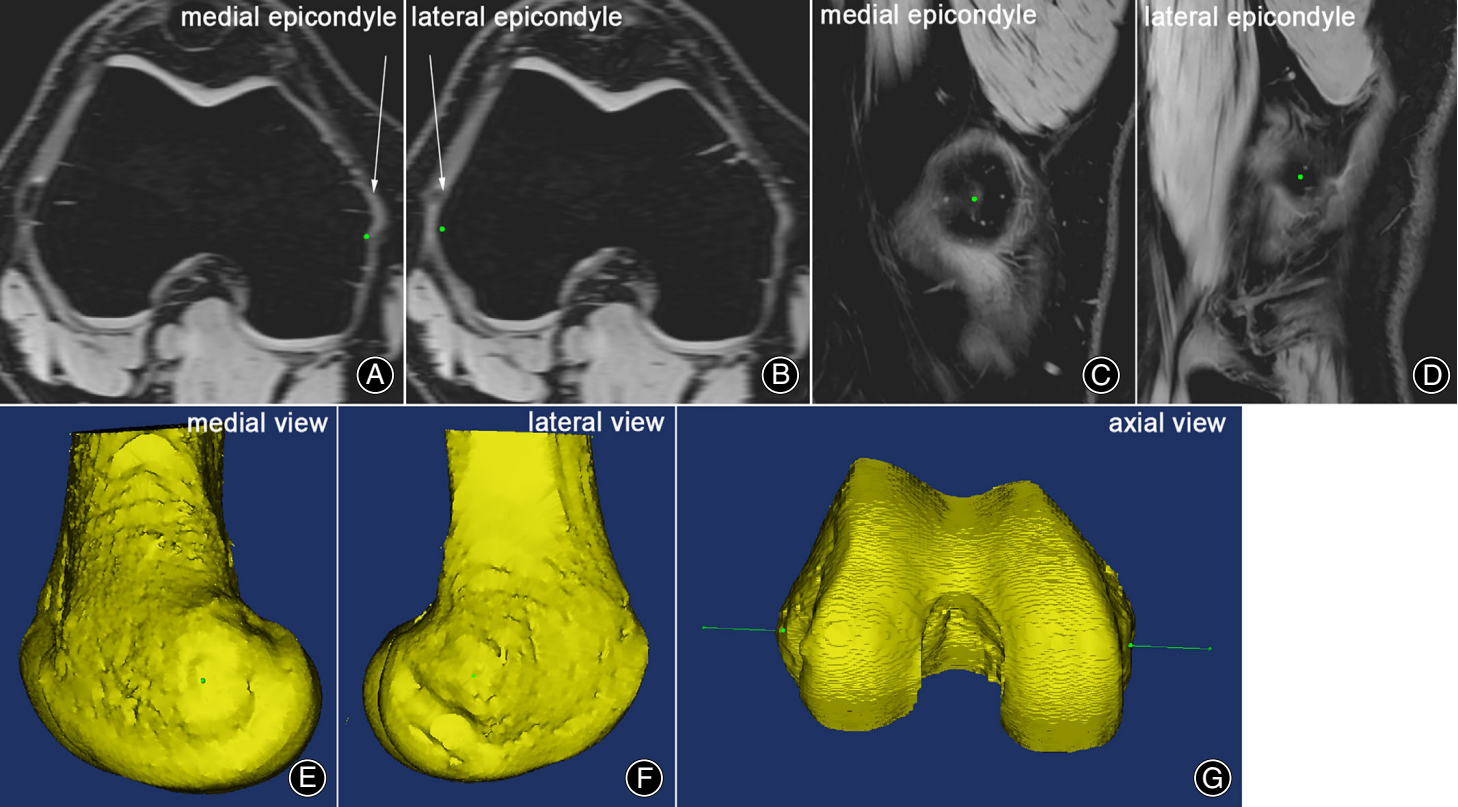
A 3D model and an MRI are used to determine the SEA. (A and B) The lateral epicondyle's most prominent point and the medial epicondyle's deepest sulcus point were both marked (the green points), and both locations were confirmed on the MRI sagittal slices (C and D). Noted that the central sulcus was surrounded by a horseshoe‐shaped bony ridge; (E and F) Both points were verified on the 3D model; (G) The green line linking the two green points was SEA.
